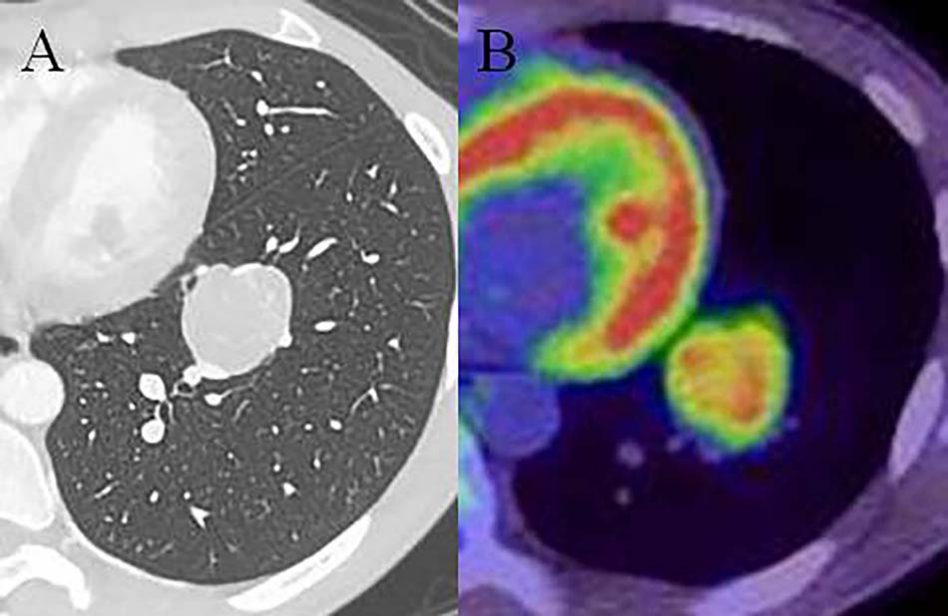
Chest computed tomography (CT) showing a 35-mm nodule in the lower lobe of the left lung (A). Fluorodeoxyglucose-positron emission tomography (FDG-PET) showing high accumulation in the nodule (SUV_max_: 5.9; B).
